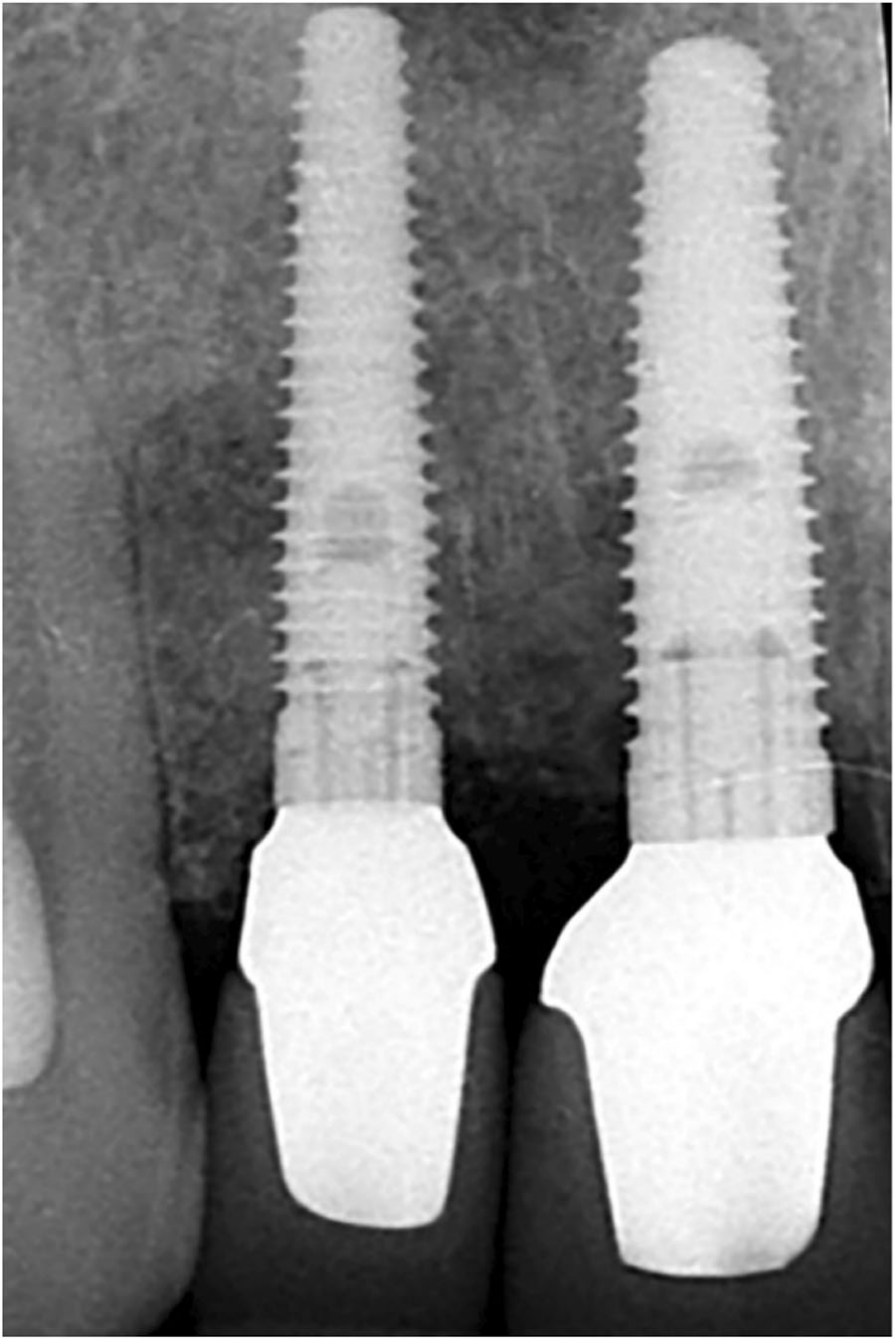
Ten‐years radiograph of two adjacent implant‐supported crowns.
